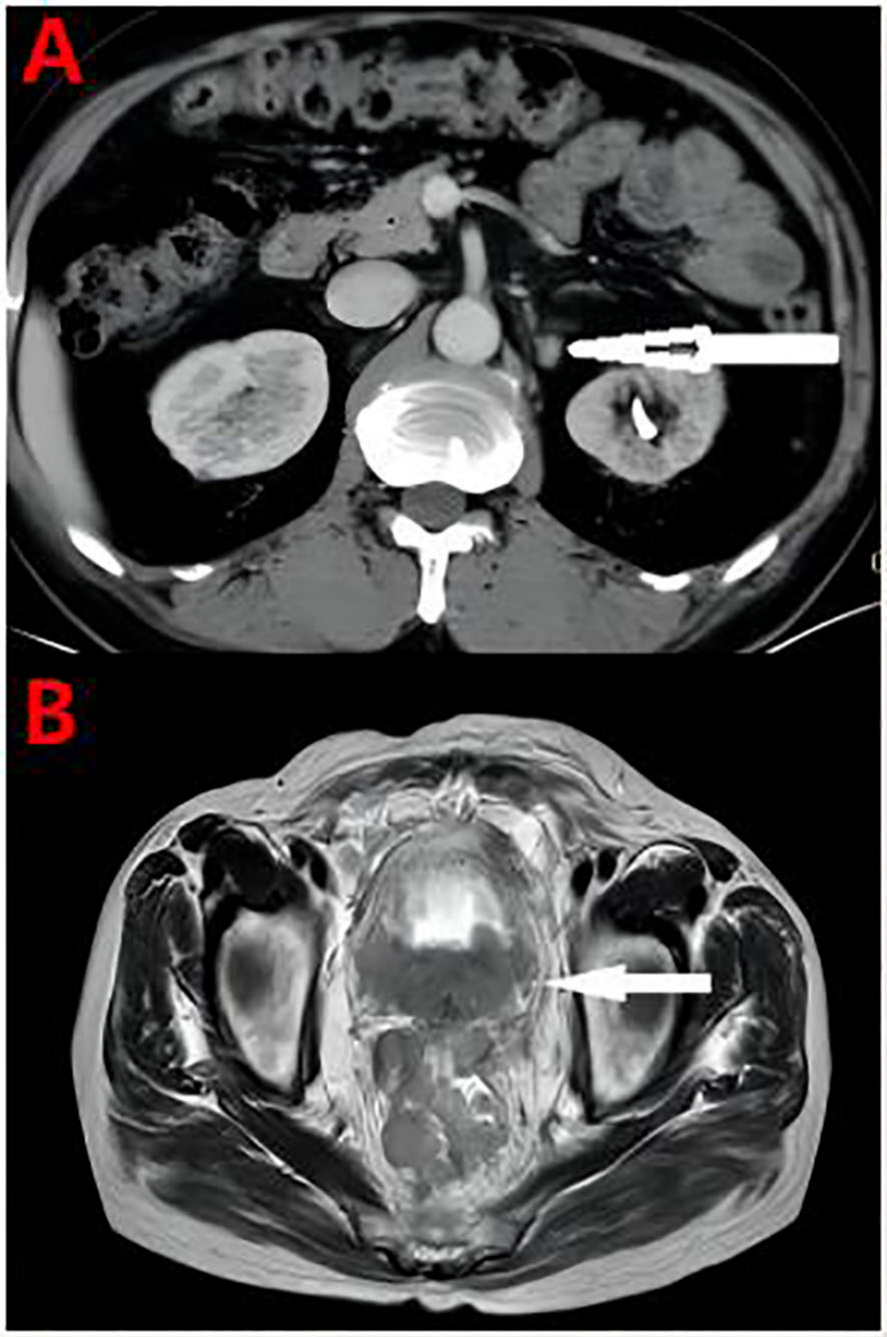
(A) At the end of the chemotherapy cycle, CT showed significant reduction of retroperitoneal lymph nodes. (B) Pelvic MRI Pelvic organ involvement and multiple pelvic lymph node metastasis were more advanced than before.
